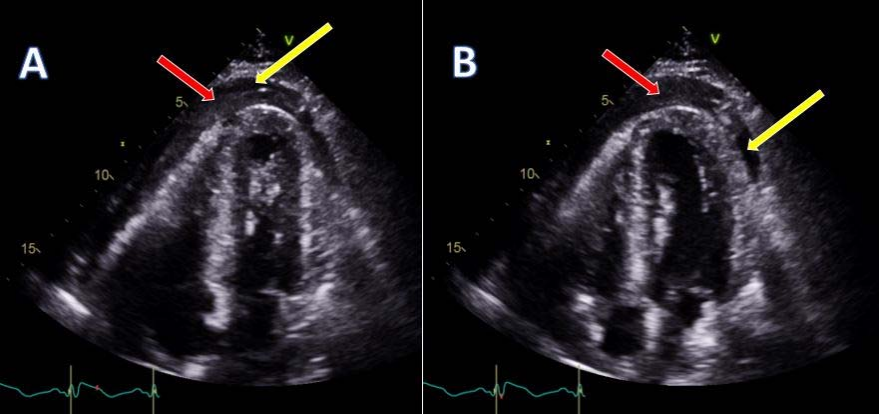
Transthoracic echocardiogram in apical 4-chamber view in (A) systole and (B) diastole. Yellow arrows point to the pericardial effusion, and red arrows point to echo-dense coagulum within the pericardial effusion.
